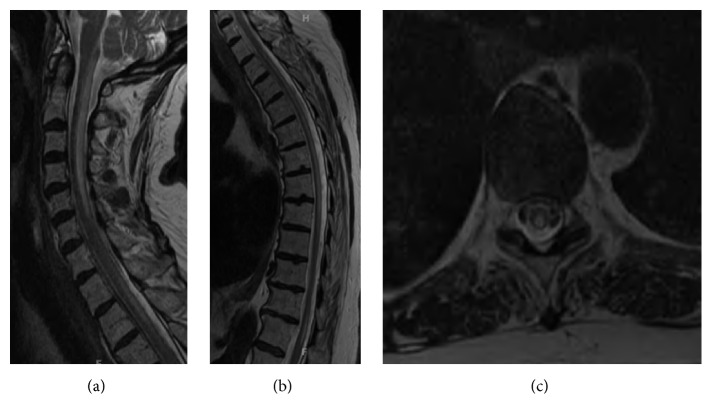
Sagittal T2-weighted MR images through the cervical (a) and thoracic (b) spine demonstrate diffuse, hyperintense signal in the posterior columns, extending nearly the entire length of the spinal cord. An axial T2W image at T9-T10 (c) demonstrates the cross-sectional extent of the signal abnormality.
